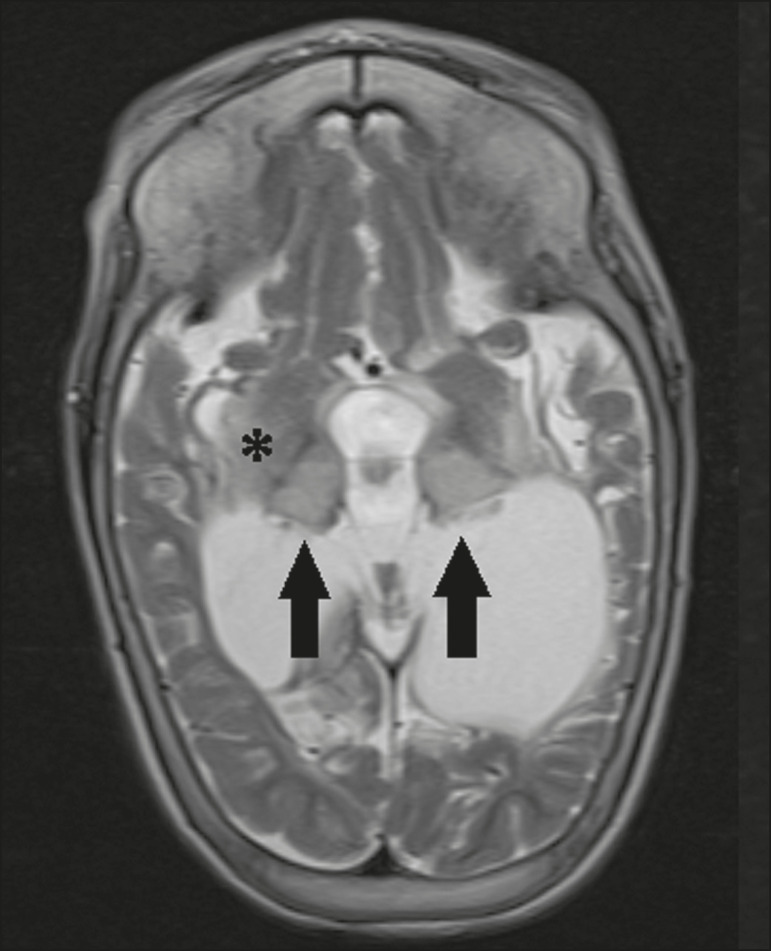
Severe hypoxic-ischemic encephalopathy. Axial T2-weighted MRI sequence showing areas of hypodensity, together with volume reductions, in the thalamus (arrows) and the nucleocapsular region (asterisk). Note also the pronounced diffuse thinning of the white matter.
